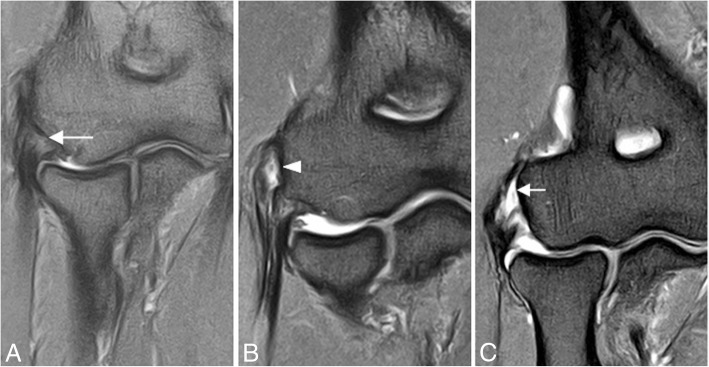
Three different patients with lateral elbow pain. Coronal FS PD-weighted MRI (a–c) showing common extensor tendinosis (long arrow), common extensor tendon partial-thickness tear (arrowhead), and common extensor tendon full-thickness tear (short arrow)
